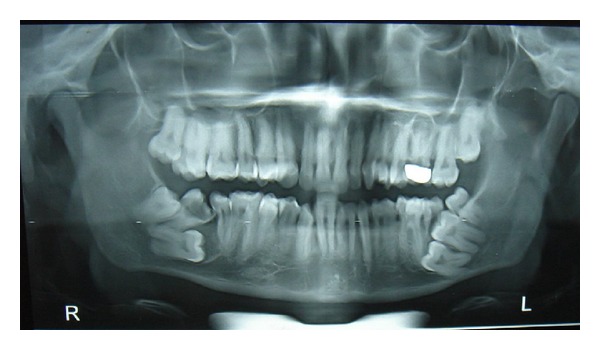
Orthopantomograph showing multiple supernumerary impacted teeth.
